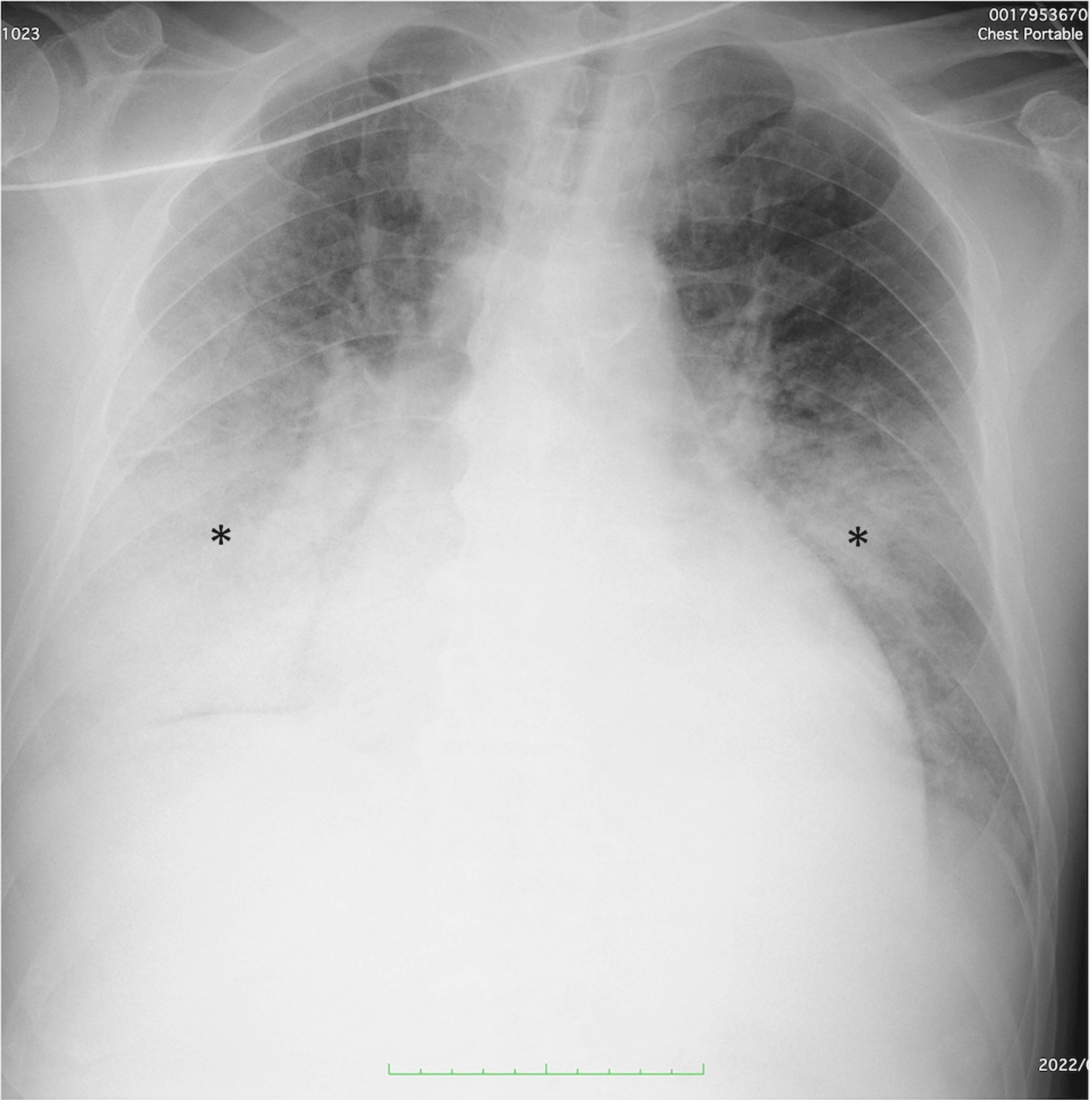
Chest X-ray on admission Chest X-ray on admission showing significant bilateral alveolar edema and pleural effusion (asterisk)
